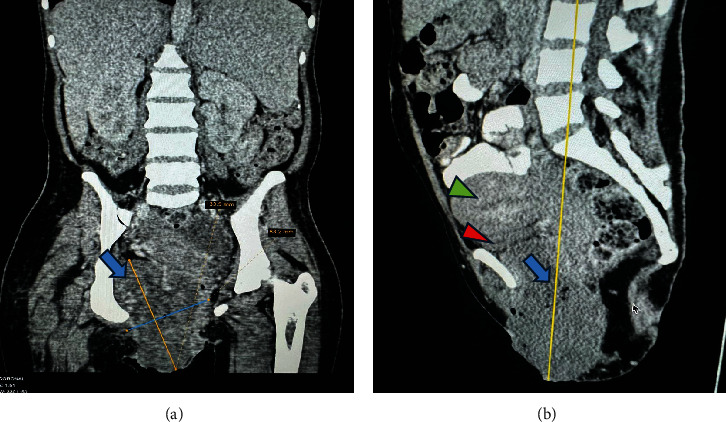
CT scan images showing a mass on the vulva. Reformatted images showing heterogenous enhancing mass on the right side of the vulva extending to the pelvis compressing the urinary bladder with no signs of infiltration and no bone involvement. (a) Coronal view, with a blue arrow showing the mass. (b) Sagittal view, showing mass (blue arrow), uterus (red arrowheads), and urinary bladder (green arrowheads).
